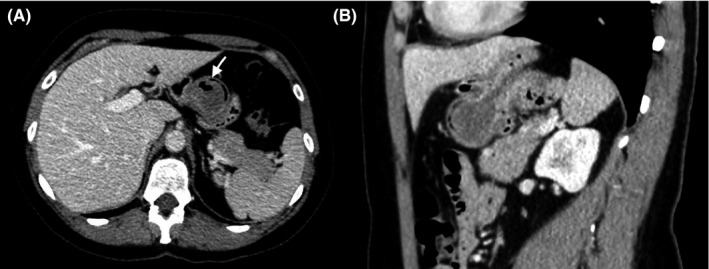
Contrast‐enhanced computed tomography of the upper abdomen. Axial image (A) showing an ulcerated mass originating from the stomach with a small air‐fluid level (arrow). Sagittal image (B) showing an organo‐axial gastrogastric intussusception
